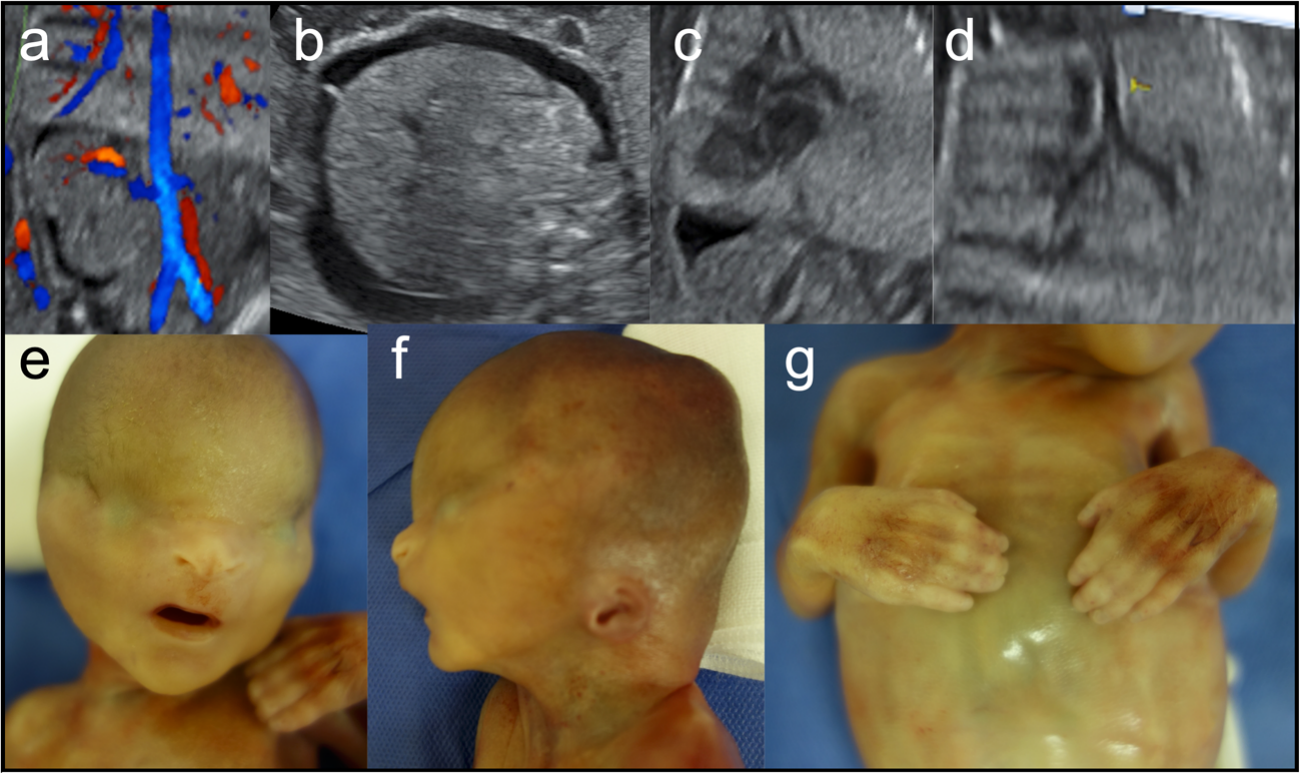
Prenatal ultrasonography images and postnatal diagnosis of a fetus with Fraser syndrome. Bilateral renal agenesis: color Doppler image of the renal artery (a). Severe ascites (b), a hyperechogenic lung with an inverted diaphragm (c), and a distended trachea (d) were observed at 19 weeks of gestation. Fetal facial features included cryptophthalmos, hypertelorism, a small, low-set nose with a bifid tip, and microtia (e, f). Bilateral cutaneous syndactyly of the hands was also observed (g).
